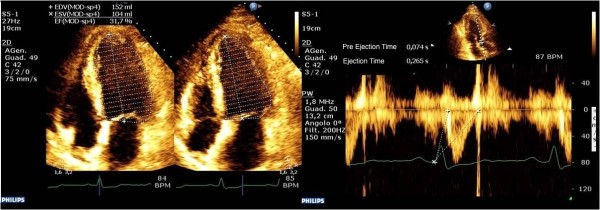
Left ventricular end-systolic elastance was calculated by using the single-beat method. These echocardiographic scans display the evaluation of ejection fraction (left image) and preejection and ejection time (right image) using aortic Doppler waveforms. Normalized ventricular elastance at arterial end-diastole (End) was measured according to the following formula:
